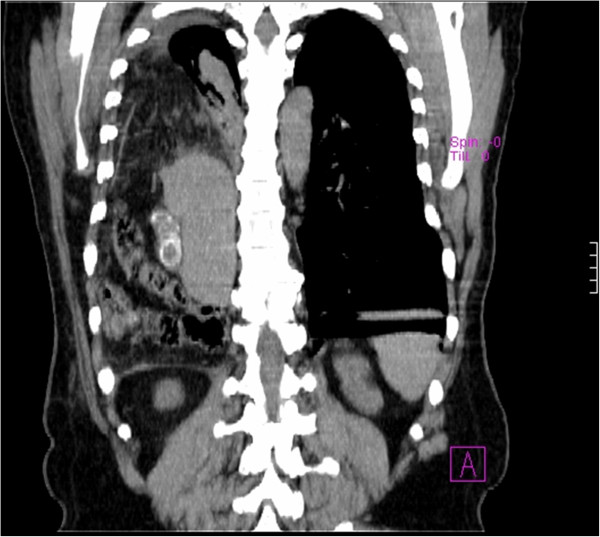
Computed tomography (CT) scan showing intrathoracal displacement of the cholecyst with three stones.
